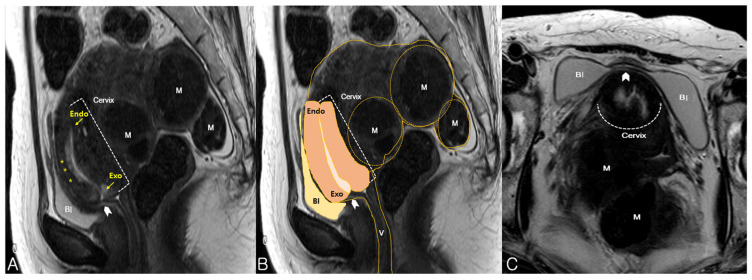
Magnetic resonance imaging findings. (A) Adenomyotic uterus with multiple myomas (M). The cervix was elongated and displaced ventrocranially, and compressed (arrowhead) the neck of the urinary bladder (Bl), as observed on mid-sagittal view; Elongated endocervical canal with high signal intensity is clearly visible with endocervical glands (asterisks); (B) A mid-sagittal view revealed an 18 × 7-cm sized, retroverted uterus with myomas (M), trapped in the pelvis. The urinary bladder (Bl) was compressed by the elongated cervix and vagina (V); (C) An axial view revealed a deformed bladder shape (arrowhead), dumbbell-shaped in this case, a clue that can be helpful in detecting an incarceration. Endo, endocervix; Exo, exocervix.
